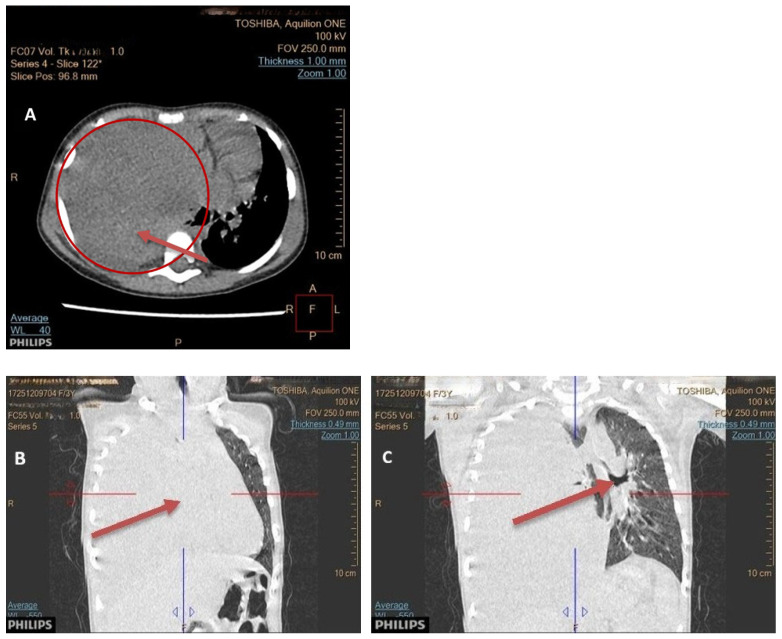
(A–C) A computed tomography imaging revealing a large, solid mass, at 11 × 9 × 11 cm, in the right hemithorax (A). The right lung is compressed and completely atelectatic (B). Marked tracheal deviation and mediastinal shift to the left side is noted (C).
